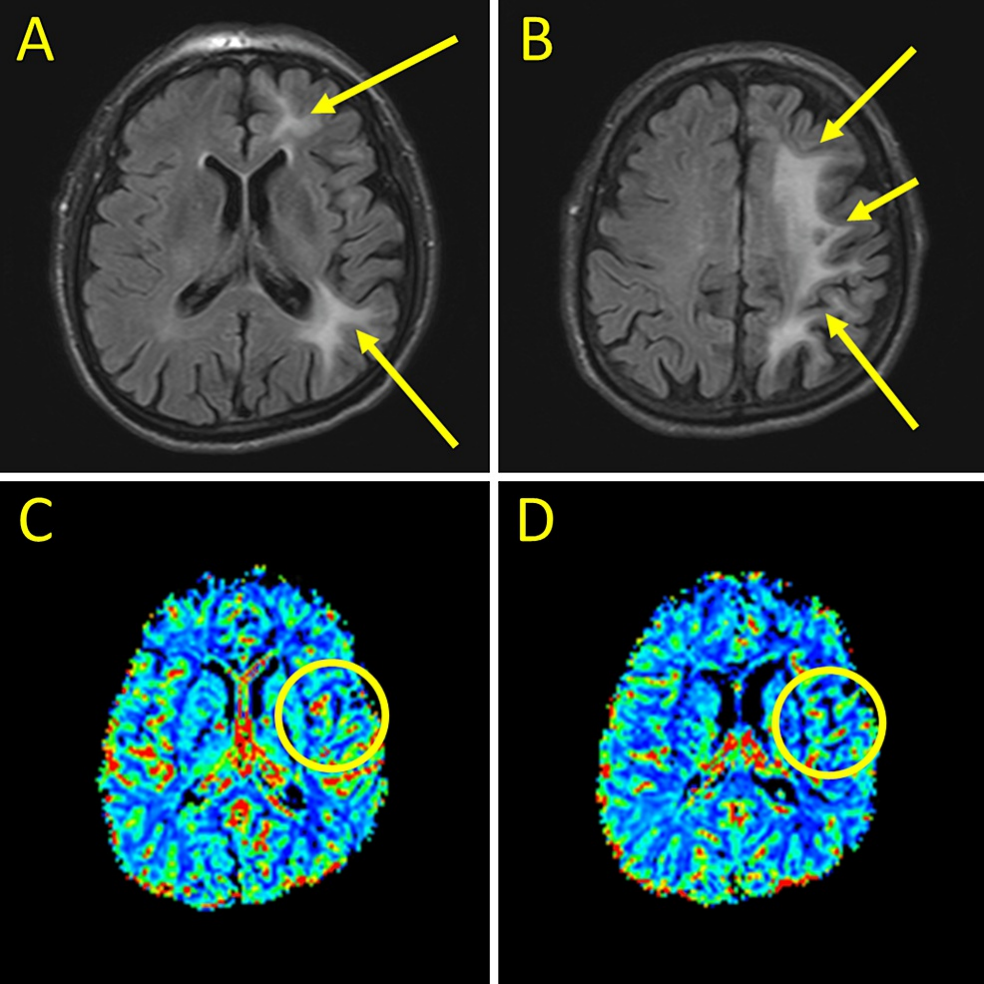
Key images from MRI brain with and without contrast and perfusion imaging (a) T2 flair post-contrast showing improvement of abnormal left-hemispheric signaling (yellow arrows). (b) T2 flair post-contrast showing abnormal left-hemispheric signaling, improved from initial study (yellow arrows). (c) MRI perfusion showing decreased cerebral blood volume compared to patient's initial study (yellow circle). (d) MRI perfusion showing persistence of decreased cerebral blood volume compared to prior study (yellow circle).
